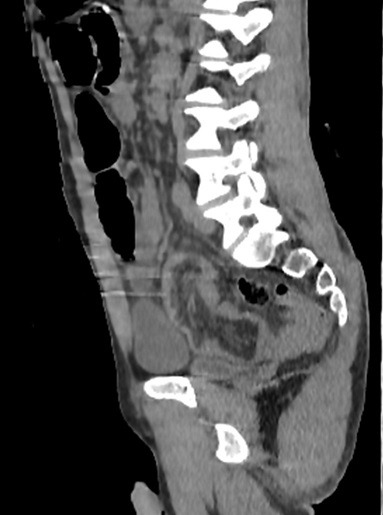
Coronal scan showing a lipoma in the wall of jejunum intestinelongitudinal reconstruction of non-enhanced abdominal CT showing the congestion of mesenteric vessels in the tortuous intestine
